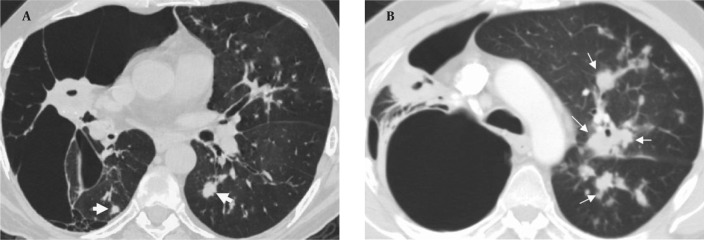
A, Axial CT image shows giant bullae in the right lung replacing normal parenchyma. Note the nodules in both lower lobes (arrows); B, Axial CT image reveals centrilobular acinar opacities (arrows) in the left upper and lower lobes which represent tuberculosis infection.
